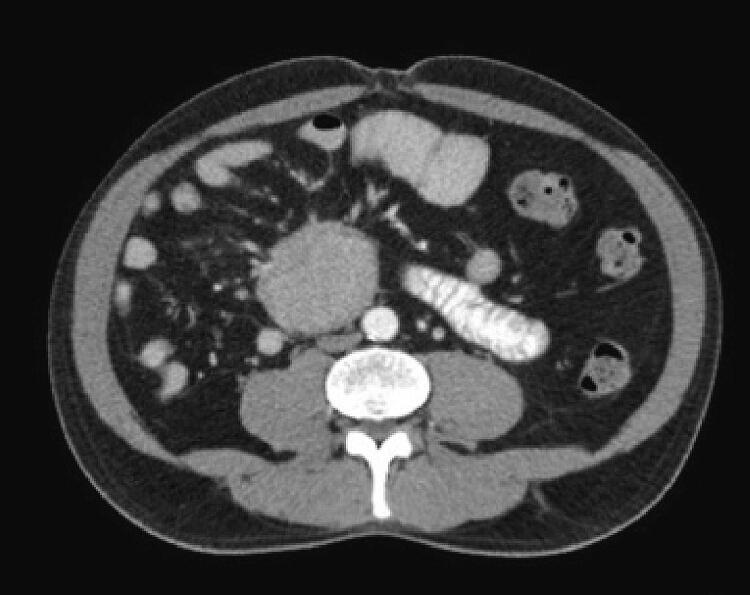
Axial contrast-enhanced abdominal CT scan section, showing a round, homogeneous, solid mass in the mesogastrium, with compressive effect on the inferior vena cava (IVC).
